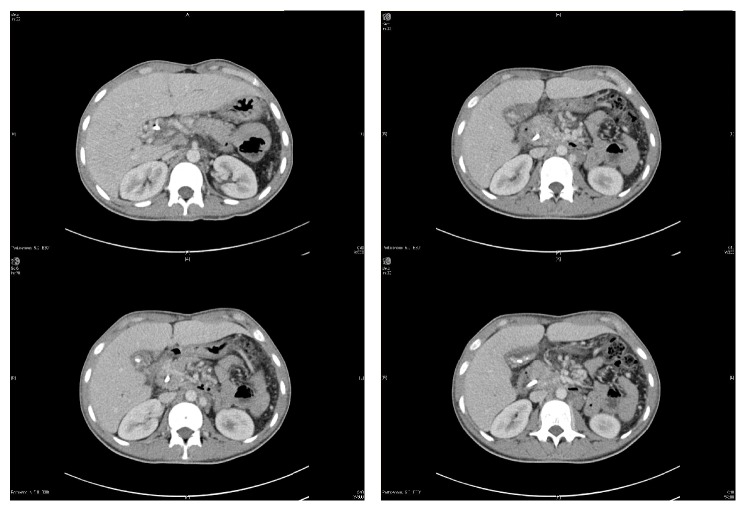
Multiple abdomen computed tomography (CT) scans showing the development of multiple collaterals in the porta hepatis replacing the portal vein, suggestive of cavernous transformation due to chronic portal vein thrombosis, in addition to multiple mesenteric and upper retroperitoneal collaterals. Multiple gallbladder stones and CBD stent are also seen. The liver is seen slightly enlarged with no detectable focal lesions. Normal enhancement of the hepatic veins. Normal size and parenchymal density of the pancreas. No detected gross masses. No detected calcification. Clear peripancreatic fat planes. Status after splenectomy.
